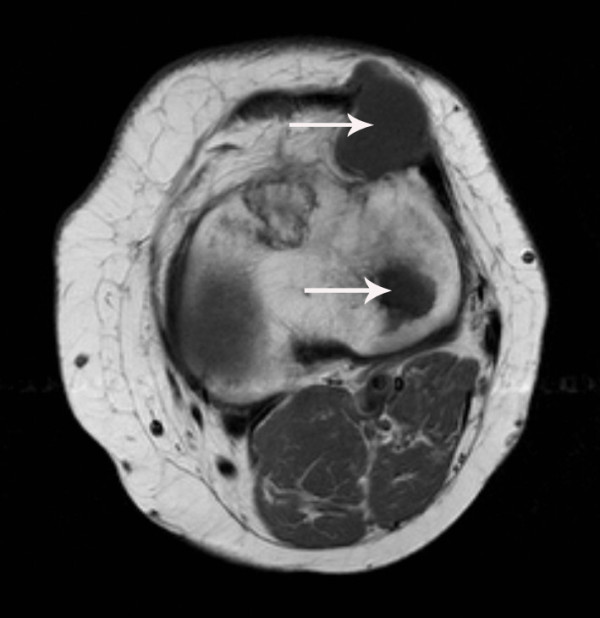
T1-weighted magnetic resonance imaging scan of our patient. Axial spin echo T1-weighted pre-contrast magnetic resonance imaging (MRI) scan of the left knee reveals two well-delineated masses (arrows) with a homogeneous low signal intensity in the lateral retinaculum and in the lateral tibia plateau.
